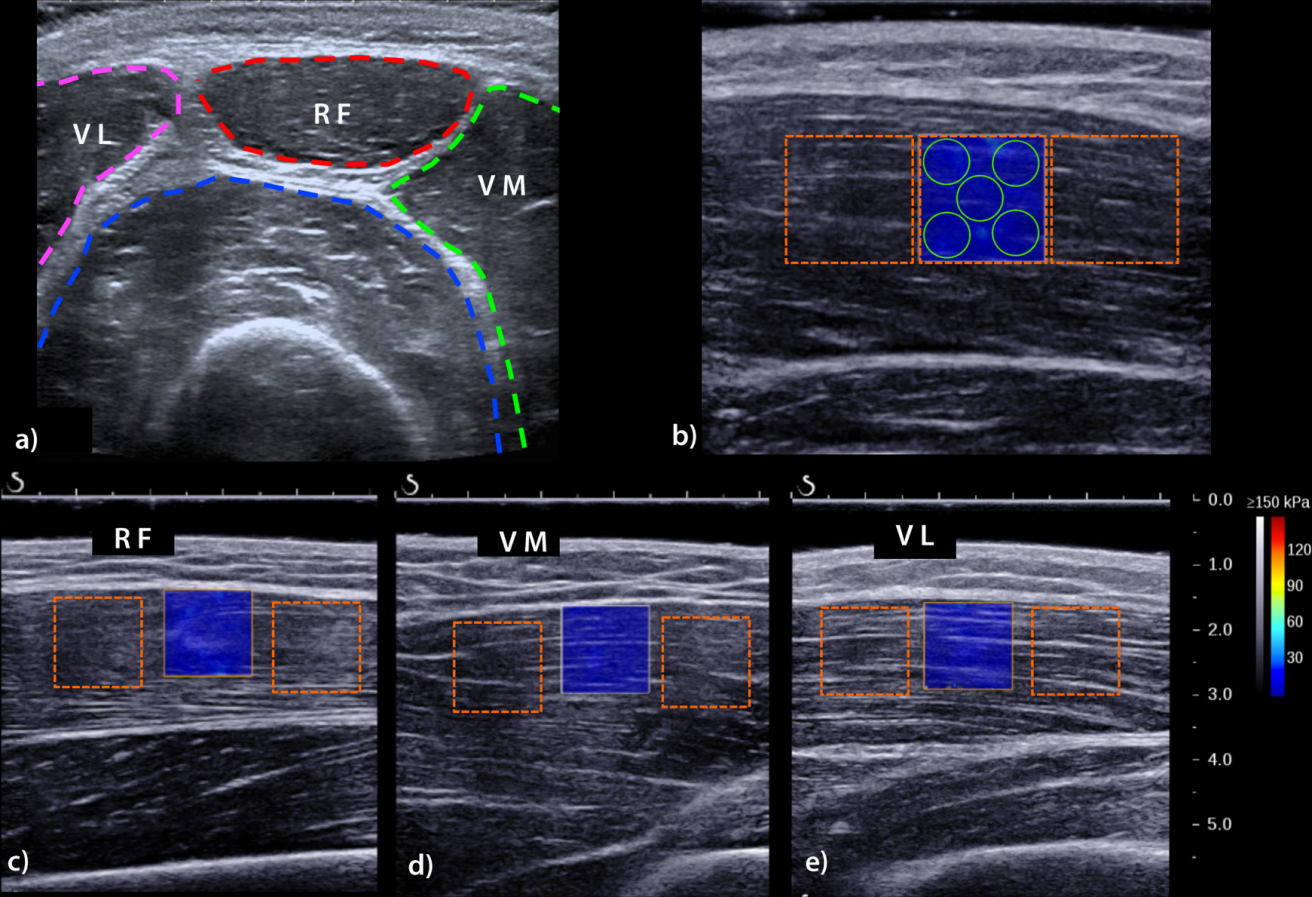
a) US image obtained in axial plane showing the different areas of interest within the quadriceps muscle: the RF, rectus femoris (red); the VL, vastus lateralis (pink); and the VM, vastus medialis (green). b) Elastographic data collection: 5 circular 5 mm-diameter ROIs (Q boxes) were manually placed within each squared SWE box by the same radiologist, who was experienced in performing musculoskeletal ultrasound. For example, this picture shows the positions of the Q boxes for the RF. The operator was blinded to the quantitative shear modulus data. c), d), E) and f) are in-plane fiber-aligned US images of the RF, VM, VL, respectively. The orange-dotted square ROIs correspond to the three sequential positions of the SWE-boxes used for the SWE measurements. Thus, at each investigation time, 9 SWE boxes were saved (3 for the RF, 3 for the VM and 3 for the VL). Note the absence of gas bubbles within the thick gel layer. The X- and Y-scales are, respectively, the in-plane and depth distances in centimeters.
